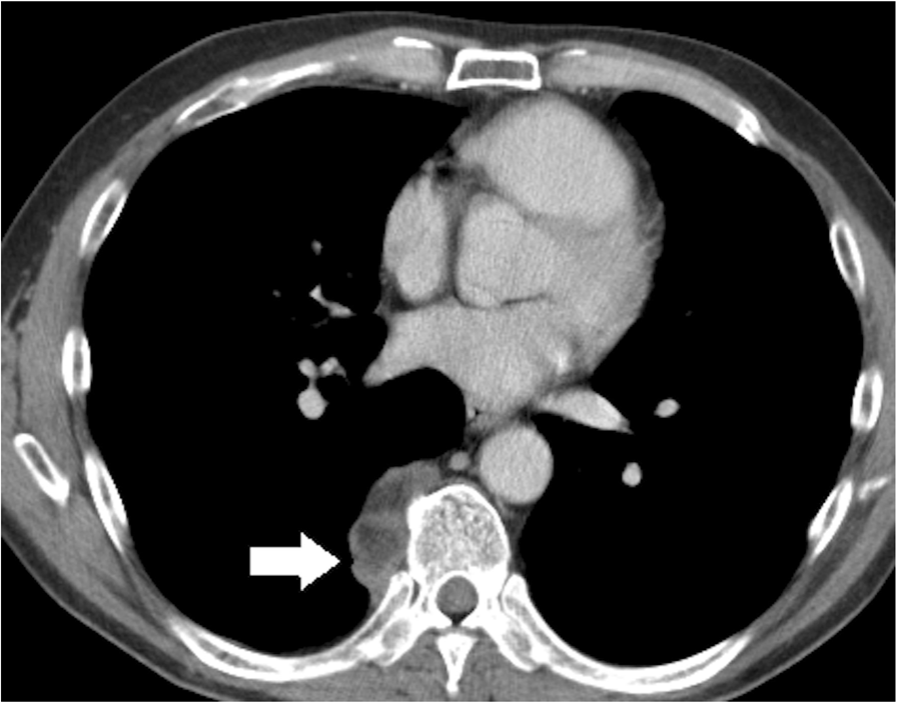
Chest CT at our hospital showed a tumor in the right posterior mediastinum (arrow). It measured 4.3 cm in diameter and was located beside the 9th thoracic vertebrae
